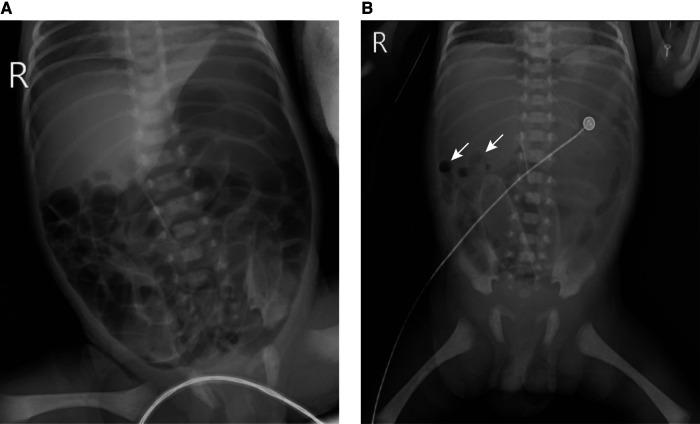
Abdominal x-ray findings of the neonatal gastric perforation before and after perforation. (A) The abdominal x-ray findings at postnatal day 4 AM before perforation; (B) the abdominal x-ray findings after perforation. The white arrow indicates pneumoperitoneum.
